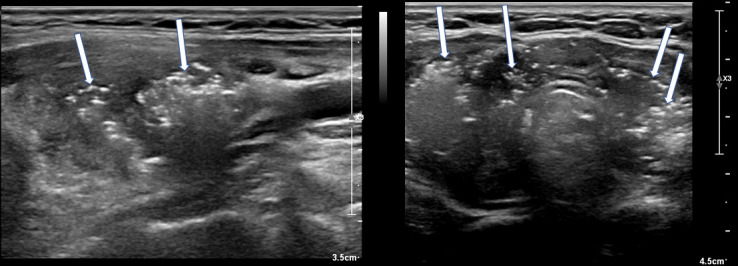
Ultrasound pattern of the thyroid lobes directly after intrathyroidal DLI. Arrows indicate characteristic snow-like effects demonstrating the spread of DLI in the affected areas.
